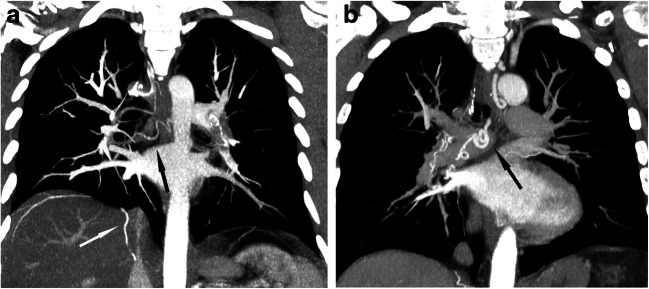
CT angiography (MIP reconstructions) shows hypertrophic bronchial arteries in both CTEPD (a) and CTEPH (b) patients (black arrows); a hypertrophic phrenic artery is also evident in (a) (white arrow)
